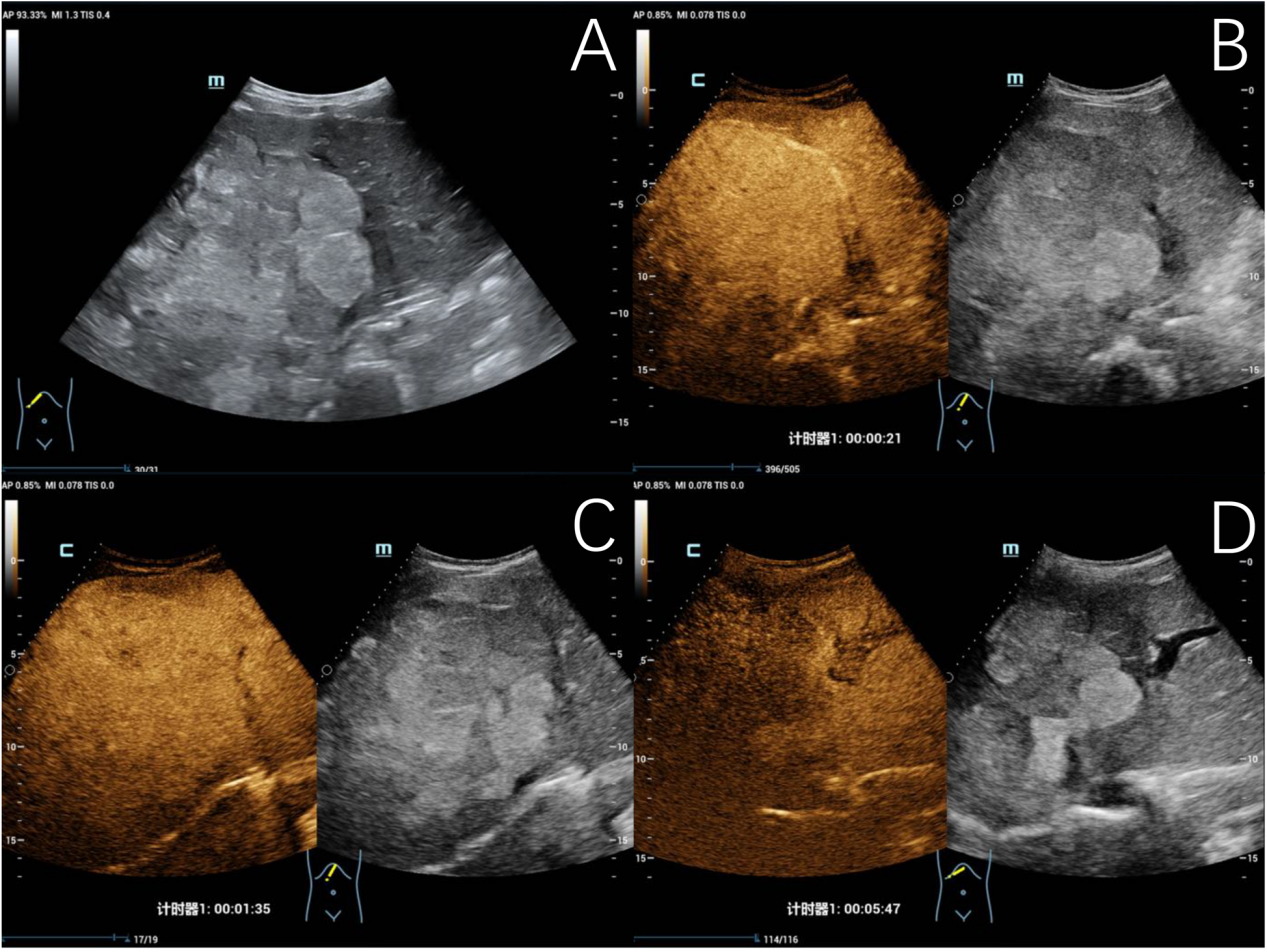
Typical ultrasound findings of hepatoblastoma (case 3). Ultrasound revealed a mixed echogenic mass in the right liver and left inner lobe, with clear boundaries, irregular morphology, and a maximum diameter of 140 mm (A) contrast-enhanced ultrasound examination showed heterogeneous hyperenhancement in the arterial phase (B), isoenhancement in the portal venous phase (C), and hypoenhancement in the late phase (D), with patchy non-enhancing areas observed within.
